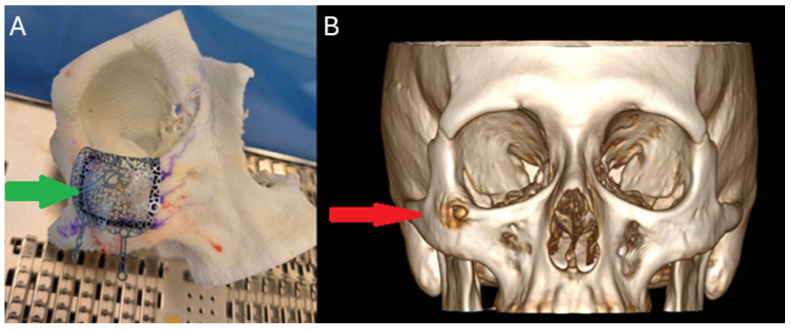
Treatment options for IH are surgical excision, sclerotherapy, radiation, or cryotherapy of the tumor [5]. During preparation for surgery authors used a 3D-printed model (A) (Form 3B, Formlabs, Somerville, MA, USA) to mark the surgical margins and establish the best approach for the patient. The creation of the 3D acrylic model was based on the location of the lesion (red arrow) in the patient’s initial CT-3D reformation (B). Intraoperatively, we used a titanium mesh (green arrow) and bent it accordingly to mimic the patient’s anatomical contours. It is worth remembering about possible alternative approaches and their indications, different than an open surgery. Radiation is rarely recommended because there is a risk of malignant transformation, and it might potentially prohibit bone growth in children [5]. Indications for radiation include increased tumor size and surgical inaccessibility for dissection. Sclerotic agents, ethanol or sodium morrhuate, can be injected into the lesion and induce an inflammatory response that shrinks the tumor or can significantly reduce its size. Sclerotherapy results in the elimination of blood vessels, but if blood flow is high, sclerotic agents might fail, compromising the results of the procedure. On the other hand, cryotherapy could also be used, but it can damage the innervation of adjacent tissues. In some cases, the lesions can be dealt with observation alone; however, some atypical swelling, pain, and other cosmetic issues for the patient are an indication for an open surgery [6]. In each case, maintaining good bone symmetry and facial features, along with a cosmetic result, is crucial given the benign nature of the tumor. Mostly, radical surgical removal is mandatory to avoid any lesion progression or recurrence. Each approach should be planned to achieve clear margins and to reduce any possible relapse of the disease. Complete surgical excision with en bloc removal of the tumor is the treatment of choice for most cases of IH. Partial excision is also an option for asymptomatic patients operated for cosmetic reasons purely, but there is a possibility for recurrence [7]. Reconstruction of the deficit can be performed by using autologous bone graft from the calvarial bone, rib, hydroxyapatite, silicone, or pedicled flaps from the infratemporal fossa, or with the use of patient-specific implants (PSI) or other solutions. The CT scan can also be used after mirroring the unaffected side to construct a perfect mirrored PSI.
